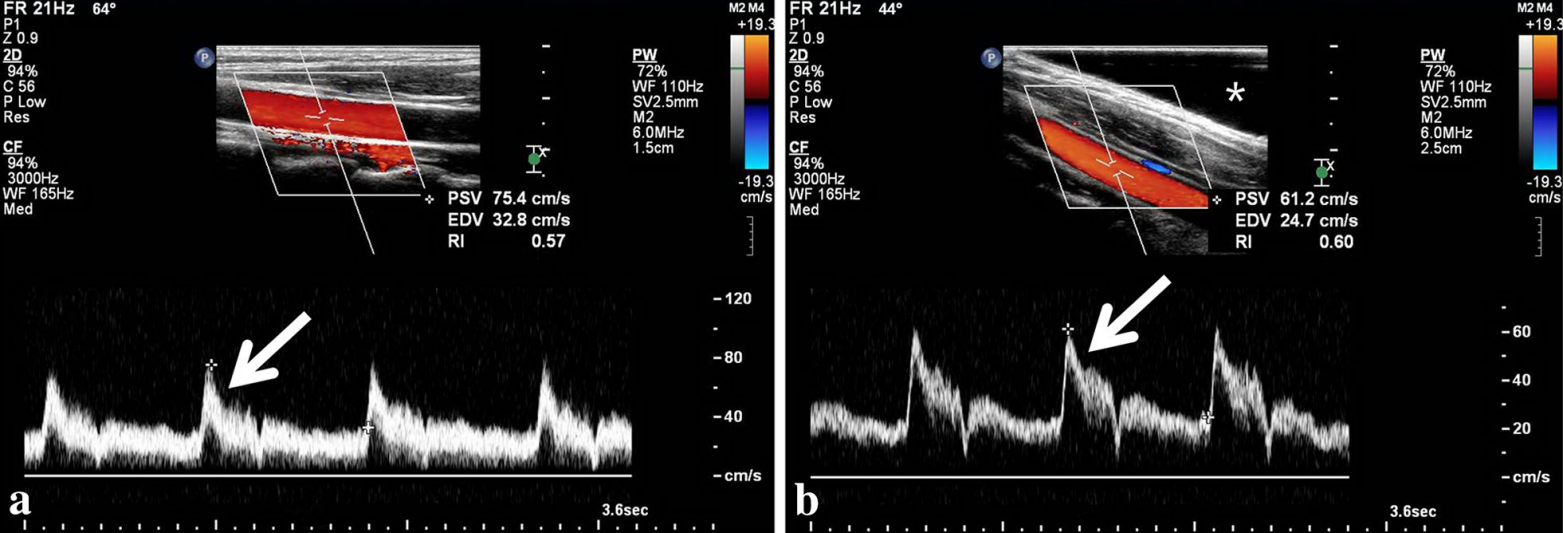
As Doppler beam-flow angles decreased, decreases in spectral broadening were seen. a Image of Doppler velocity measurement taken at a 64° beam-flow angle without the use of slanted gel pads shows spectral broadening throughout nearly the whole velocity range (*arrow*). b Image of Doppler velocity measurement taken at a 44° beam-flow angle with the use of a slanted gel pad (*star*) shows much decreased spectral broadening seen mostly throughout only the upper velocity range (*arrow*)
